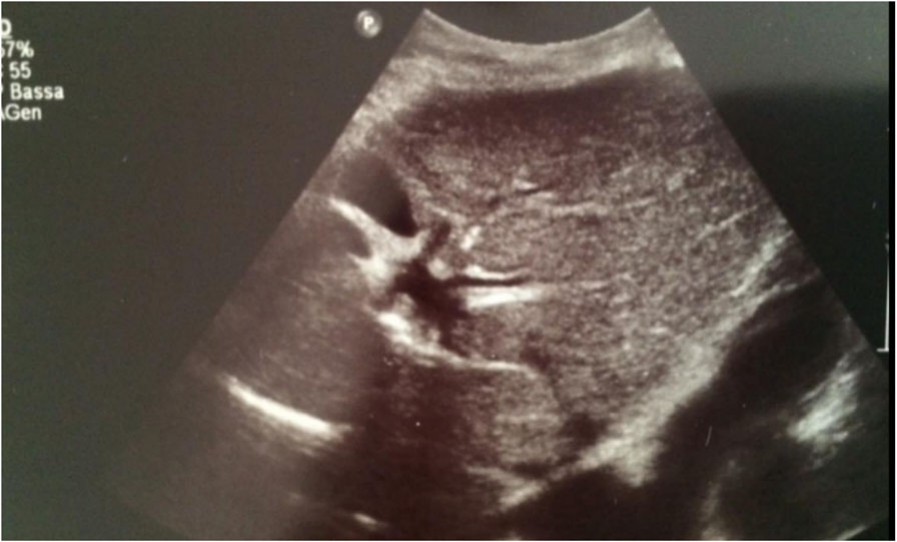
Liver ultrasound examination performed 10 months after the end of treatment when AFP was 10 ng/ml. No signs of liver cirrhosis and no signs of tumor were detectable
